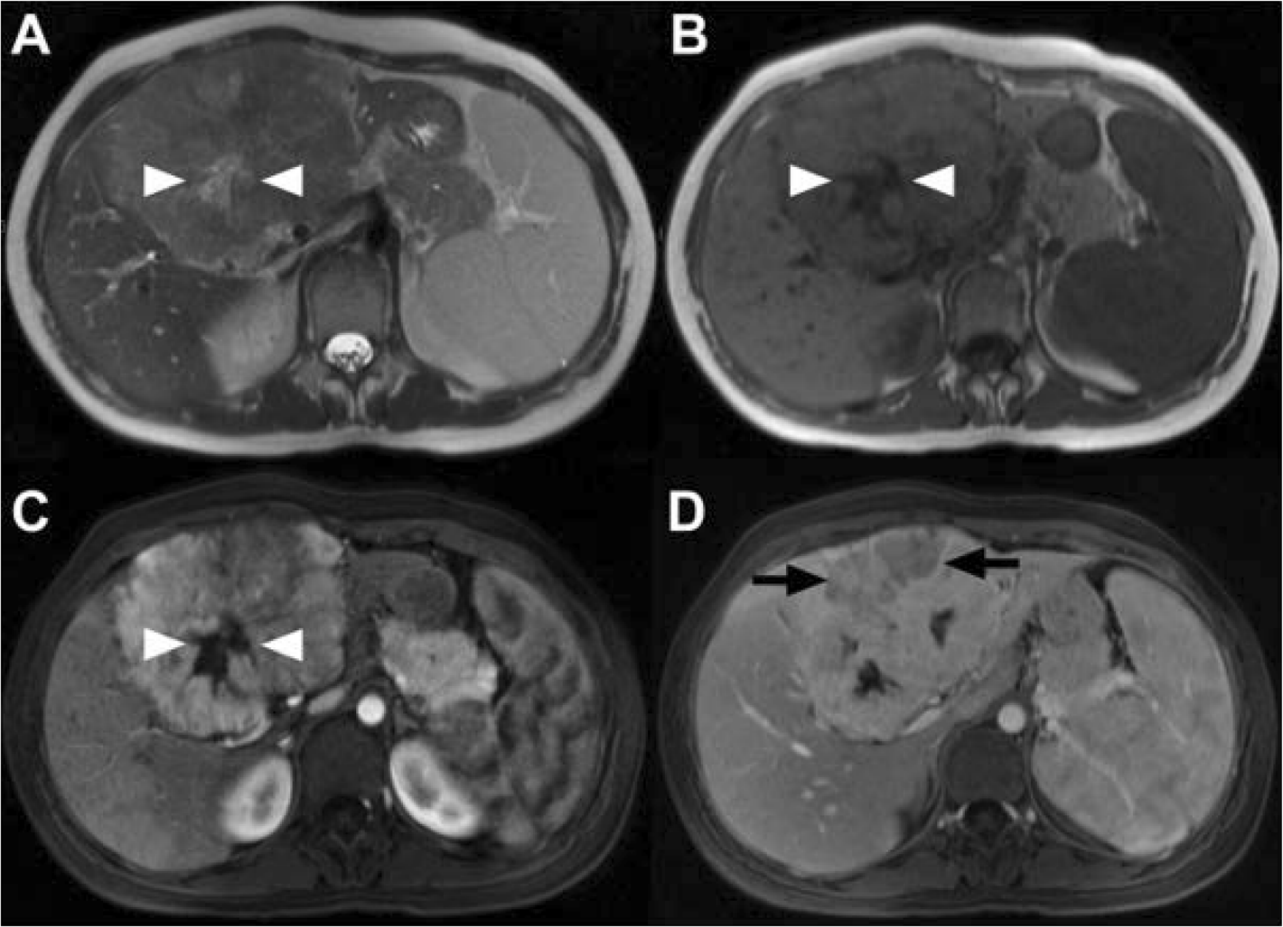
MRI scan of a 17-year-old female patient. a) Axial T2 weighted Half-Fourier Acquisition Single-shot Turbo spin Echo (HASTE) sequence shows a large inhomogenous hepatic lesion with a central moderately T2-hyperintense scar (white arrowheads) in the left lateral and medial as well as right anterior sectors. b) The central scar (white arrowheads) is more prominent in the axial native T1 Fast Low-Angle Shot (Flash) 2D sequence. c) The lesion shows markedly inhomogenous hyperenhancement in the arterial phase (axial T1 FLASH 3D). The central scar (white arrowheads) does not enhance. d) A ventral part of the lesion shows washout appearance (black arrows) in the portal venous phase (axial T1 FLASH 3D).
